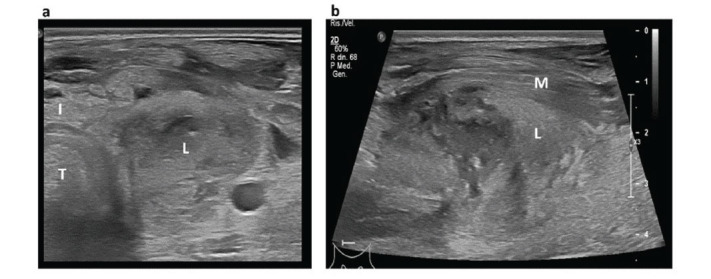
Thyroid ultrasound at time of admission, both in transversal (a) and longitudinal section (b). An inhomogeneous hypoechoic area with colliquated areas and poorly defined margins can be observed in the left thyroid lobe, which extends to the anterior neck muscles. T=trachea, I: thyroid isthmus, L=left thyroid lobe. M=muscle.
